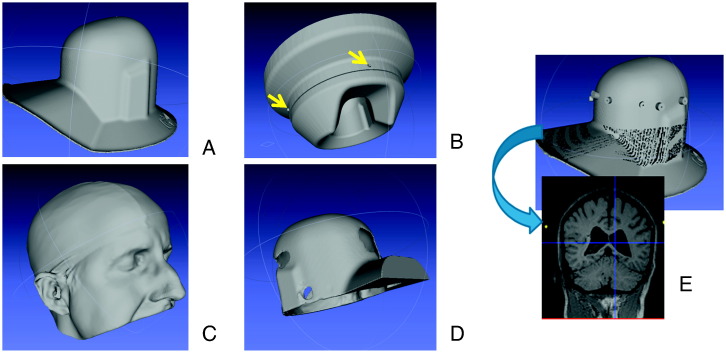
Head cast/coregistration.* Panel A shows the optical scan of the dewar helmet, which, in combination with an optical scan of the subject's head (Panel C), serves as the basis for creating the template of the head cast (Panel D). For coregistration purposes, MRI visible markers are placed on the outside of the dewar helmet, such that their position in an MRI structural image can be related to its counterpart in an optical scan (Panel E). To obtain a transform which allows us to relate MEG sensor space and MRI space, we use the 4 measurement coil positions in relation to the MEG system dewar in a manufacturer supplied image (Panel B).
